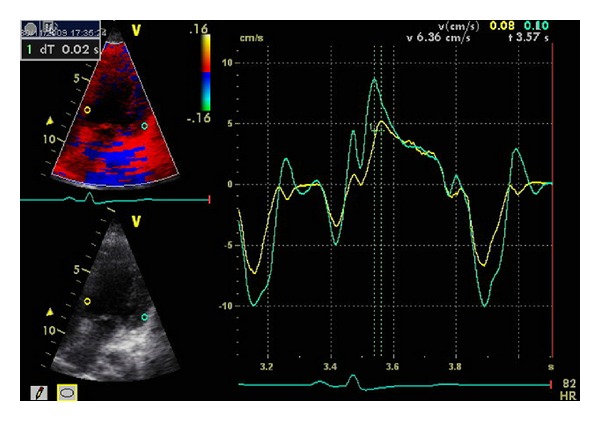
Determination of time-velocity curves using color-coded tissue Doppler and Q-analyses (from left ventricular septal and lateral wall sample points selected in an apical long-axis view). In this example (patient no. 6), no significant delay is demonstrated between septal and lateral left ventricular walls following catheter ablation.
